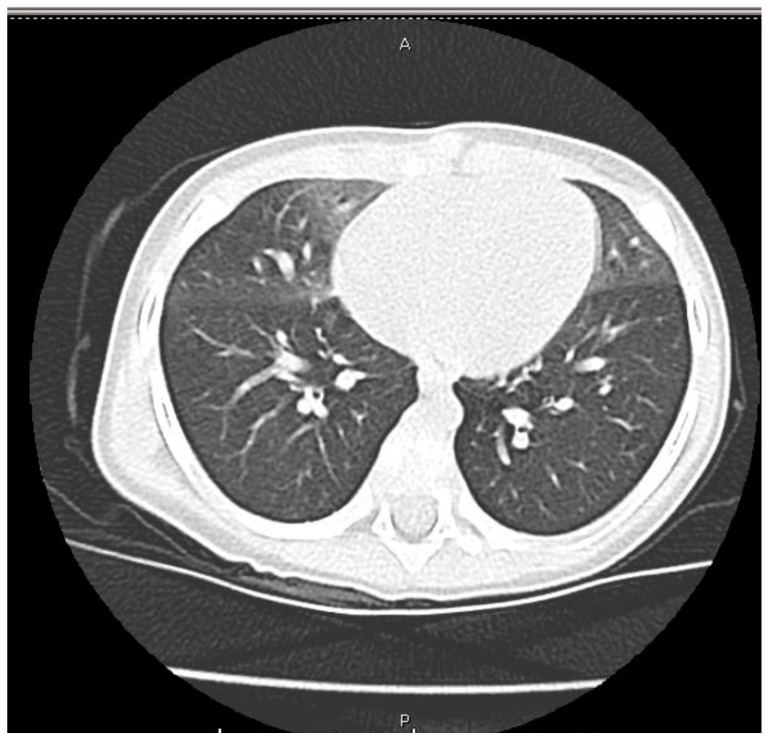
High-resolution chest computed tomography (CT) documenting patchy areas of ground-glass opacity involving the right upper lobe, middle lobe, and lingula, as well as mosaic areas of air-trapping, which are suggestive of a diagnosis of neuroendocrine cell hyperplasia of infancy (NEHI). A: anterior, P: posterior.
